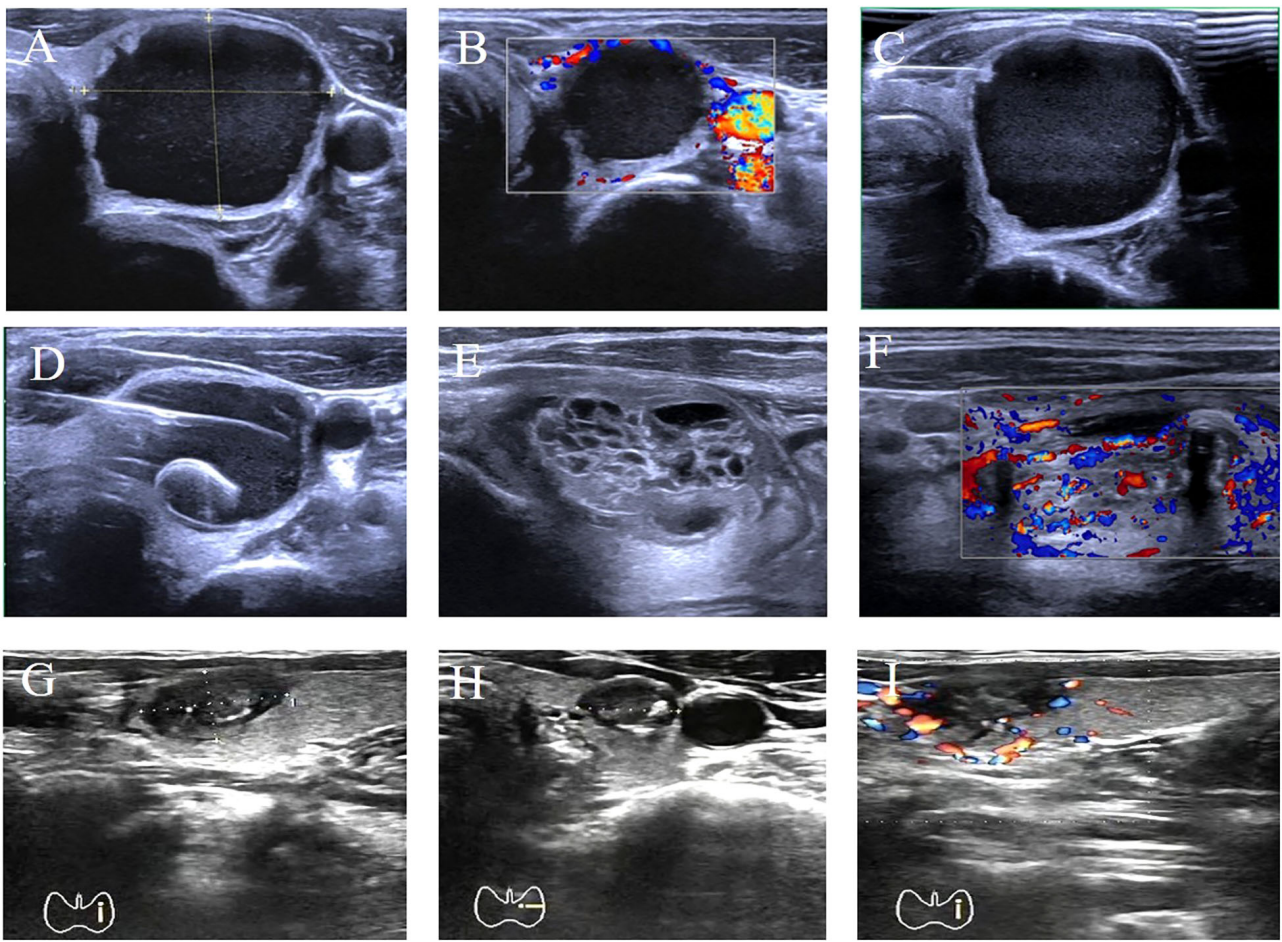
Comparison of preoperative and postoperative results in one patient. (A) Transverse plane ultrasound showing a 35×30×23 mm cystic-solid mass (predominantly cystic) in the left thyroid lobe. (B) Color Doppler ultrasound demonstrating blood flow signals around the lower pole of the nodule. (C) Longitudinal plane ultrasound during fine-needle aspiration biopsy (FNA) of the nodule. (D) Transverse plane ultrasound showing placement of a single-lumen pigtail catheter into the nodule. (E, F) Longitudinal plane ultrasounds on postoperative day 2 revealing full expansion of the solid component, thickened cyst wall, and appearance of blood flow signals. (G–I) Transverse plane ultrasound images at different levels obtained 3 months post-ablation. Ultrasound Imaging Planes: (A) Transverse; (B) Color Doppler; (C) Longitudinal (FNA); (D) Transverse (catheter placement); (E, F) Longitudinal; (G–I) Transverse (post-ablation). Post-ablation nodule size: 13.6×8.6×9 mm. Volume Reduction Rate (VRR): 95.8%.
